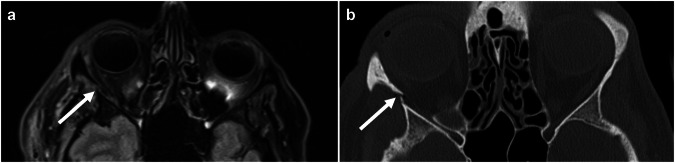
Axial plane of a fracture of the right lateral orbital wall (white arrows). a Fluid attenuated inversion recovery (FLAIR) image (axial MRI section; 3 mm thick) depicting the slightly dislocated fracture. No associated hematoma is found. b CT of the same patient showing the same fracture morphology
